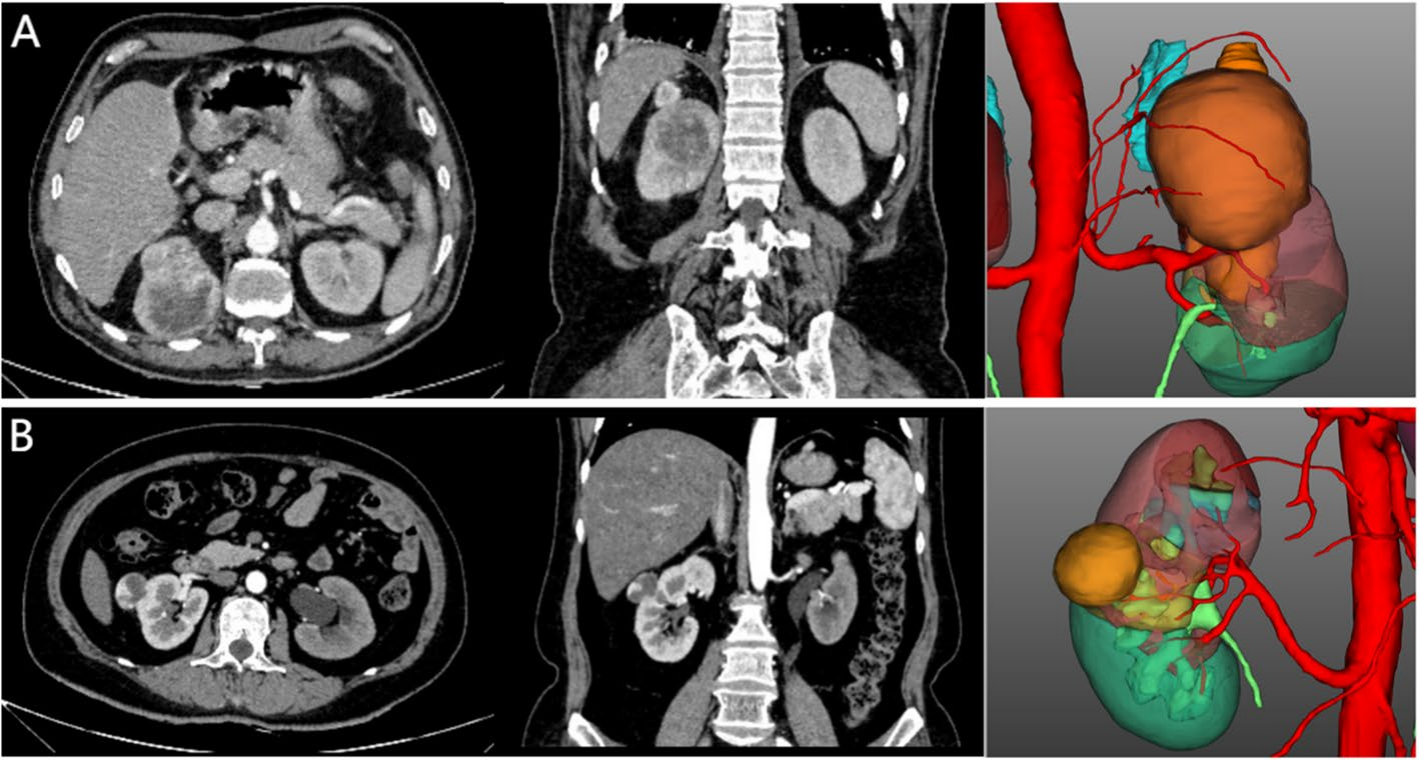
Representative CT images and reconstructed 3D models for one male patient and one female patient. Legends: A Male, 70 years old, right renal tumor, the maximum tumor diameter was 7.5 cm, there were 2 ARA and 1 branch of renal artery before the renal hilum. B Female, 50 years old, right renal tumor, the maximum tumor diameter was 3.0 cm, there is 1 ARA
